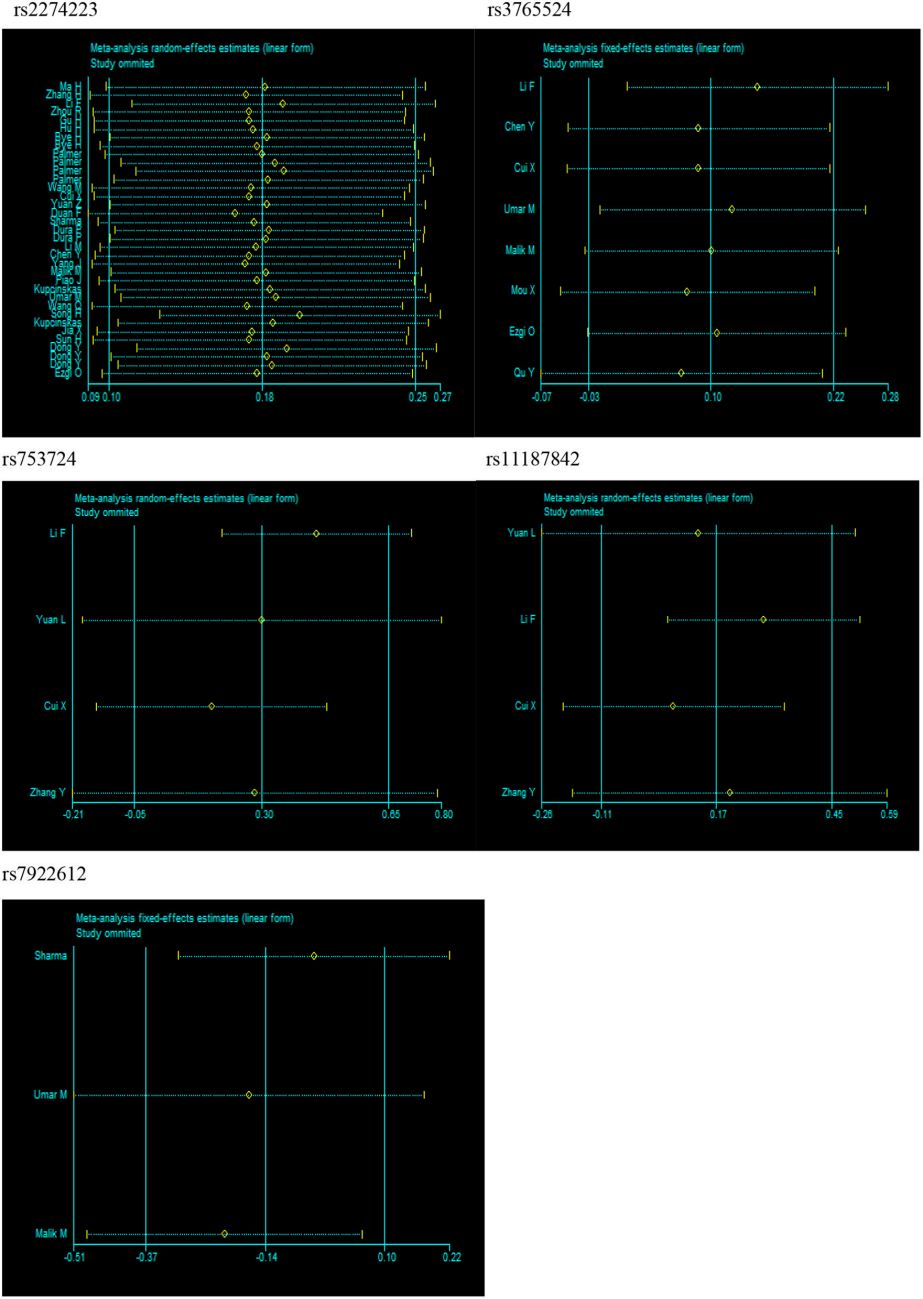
Sensitivity analysis for *PLCE1* polymorphisms and cancer risk in dominant model (rs2274223: AG+GG vs. AA; rs3765524: CT+TT vs. CC; rs753724: GT+TT vs. GG; rs11187842: CT+TT vs. CC; rs7922612: CT+TT vs. CC).
